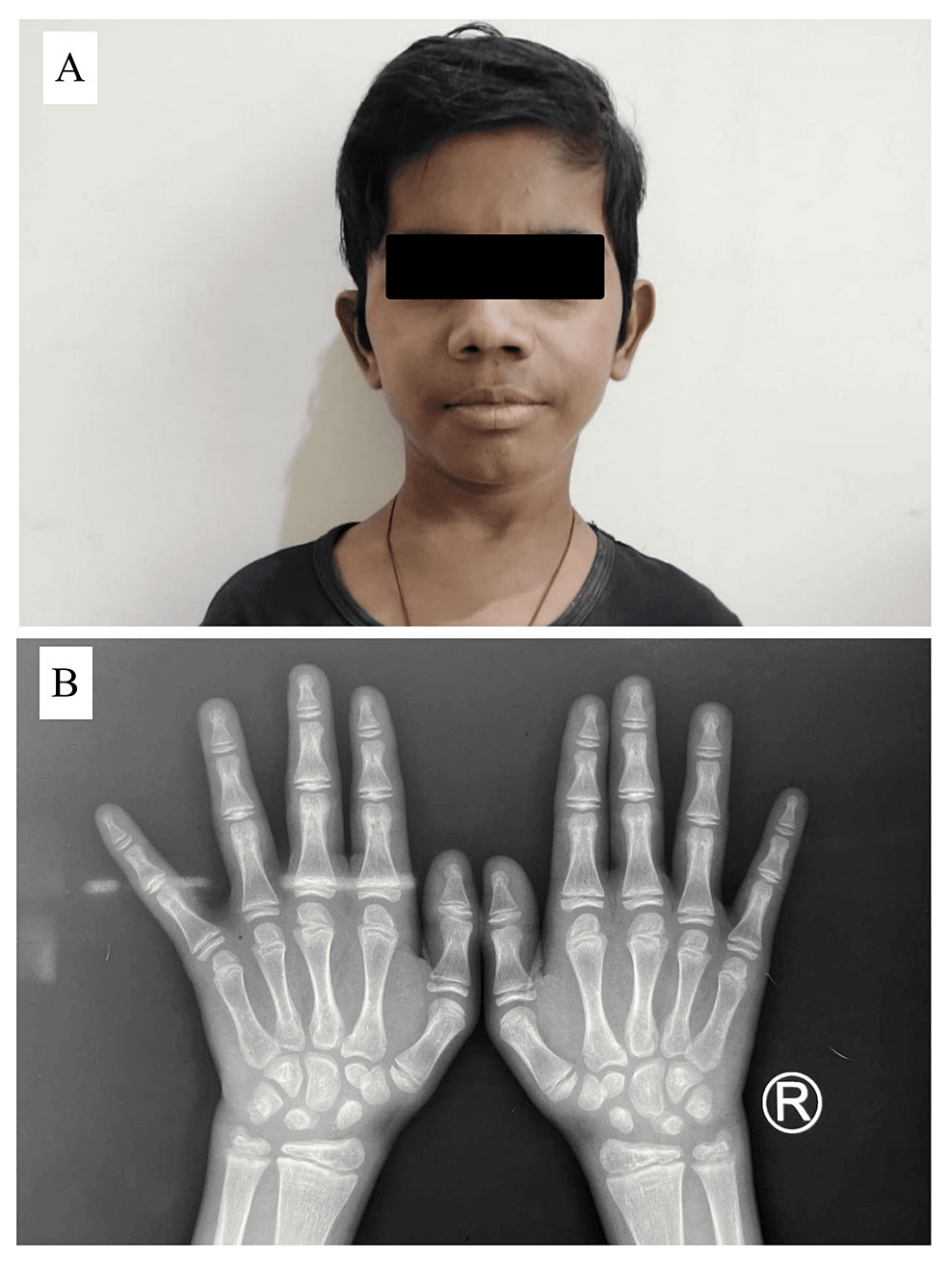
(A) Resolution of myxoedematous changes. (B) Post-treatment radiograph matches the reference bone age of an eight-year-old boy.
